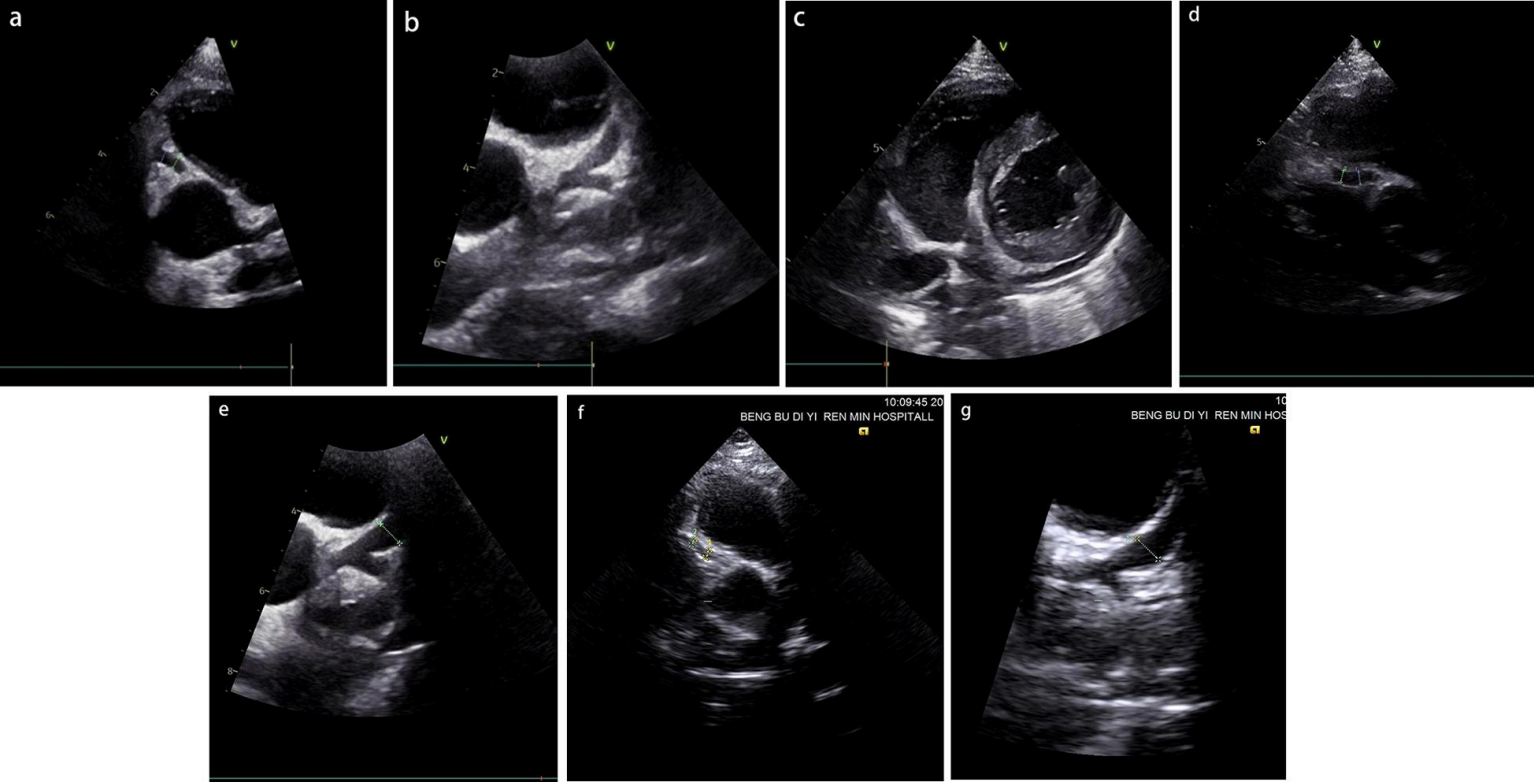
(a–c) The results of the patient's initial coronary artery echocardiography following hospital admission (on day 12 of illness). (d,e) The findings obtained prior to discharge (on day 33 of illness). (f,g) The results from the most recent follow-up evaluation (6 months after the onset of illness). RCA dilation with a diameter of 3.5 mm (a), left coronary artery dilation with LMCA 4.0 mm, left ascending artery (LAD) 4.4 mm, LCX 3.2 mm (b), and mild pericardial effusion (c) were shown. RCA dilation with a diameter of 4.3 mm (d), left coronary artery dilation with LMCA 3.7 mm, LAD 6.3 mm, LCX 4.3 mm (e), RCA dilation with a diameter of 2.7 mm (f), left coronary artery dilation with LMCA 3.2 mm, LAD 5.0 mm, LCX 2.6 mm (g).
